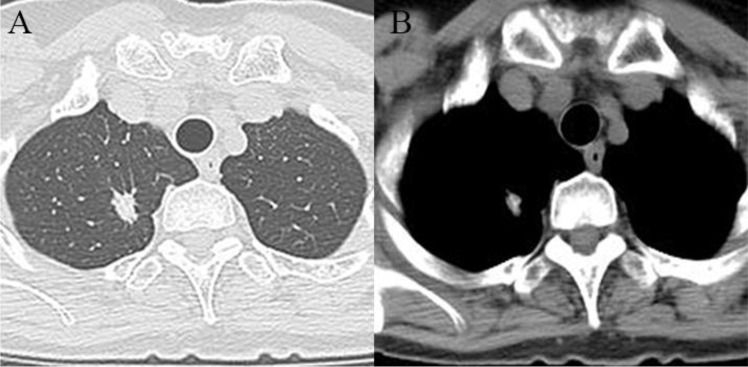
Chest CT scan on October 27, 2022. (A) Lung window; (B) Mediastinal window. showing an irregular solid nodule in the right upper lobe, 1.5cm × 0.8cm.
